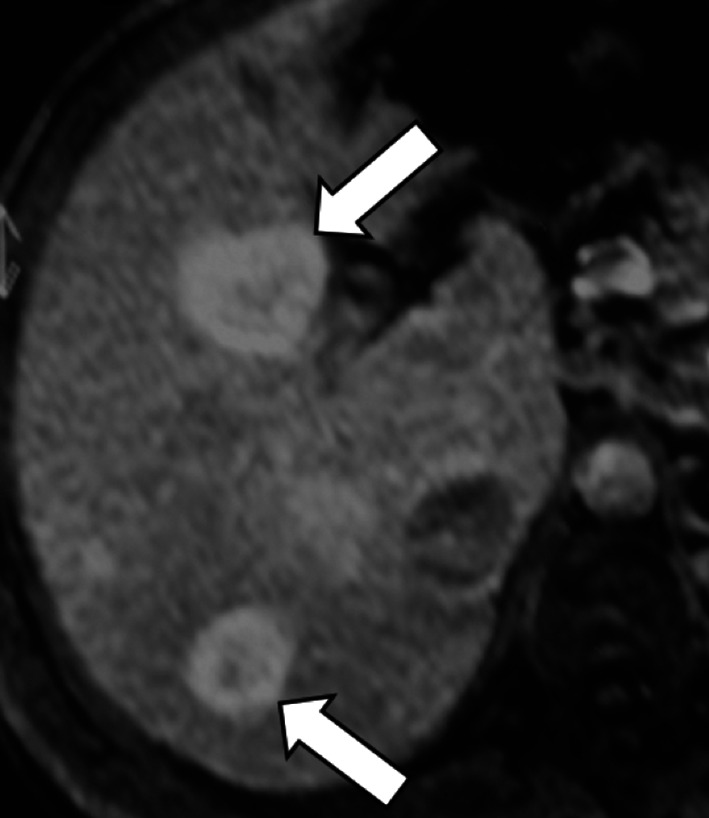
A 41-year-old man with cavernous transformation of the portal vein and FNH-like nodules. Gadoxetate disodium-enhanced MRI shows two FNH-like nodules (arrows) that are hyperintense in the hepatobiliary phase with central small hypointensity due to a central scar
